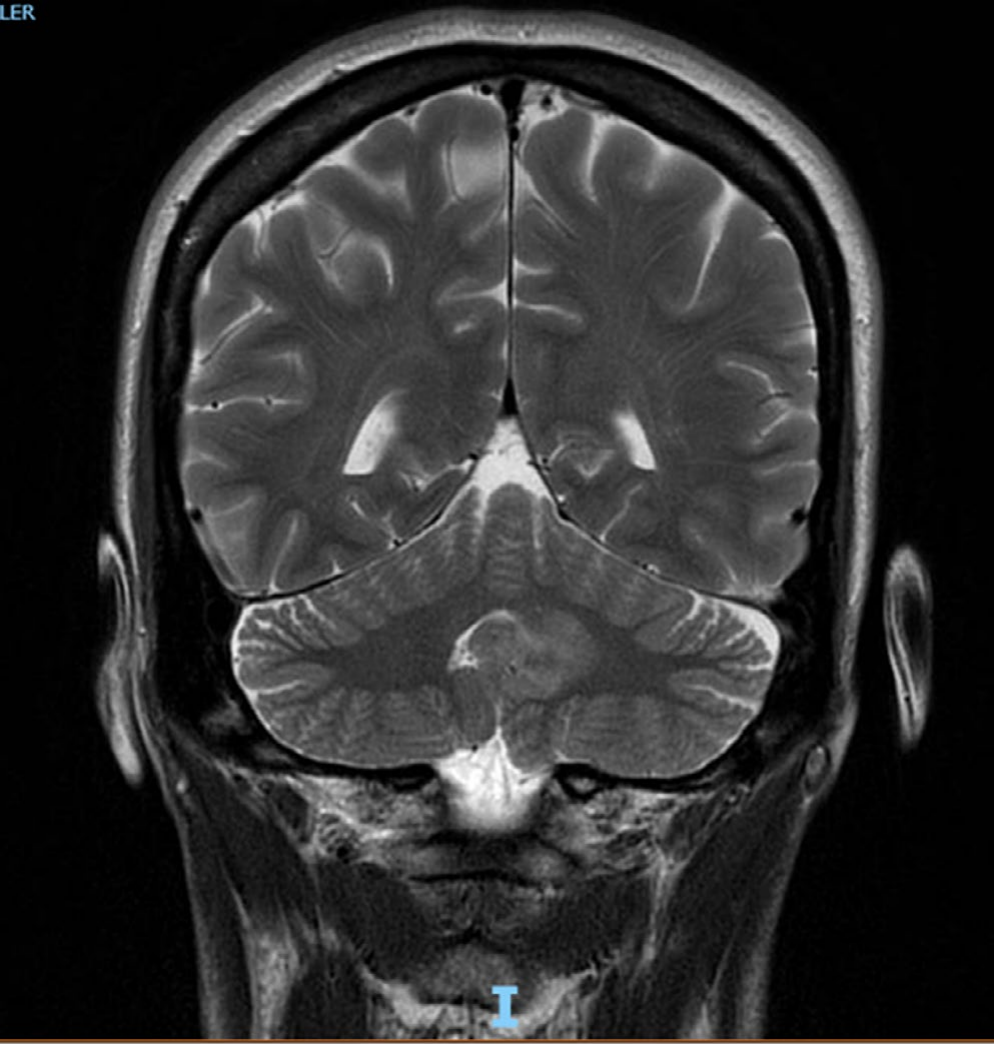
MRI show a hyperintense left cerebellar focal lesion on T2‐weighted imaging
